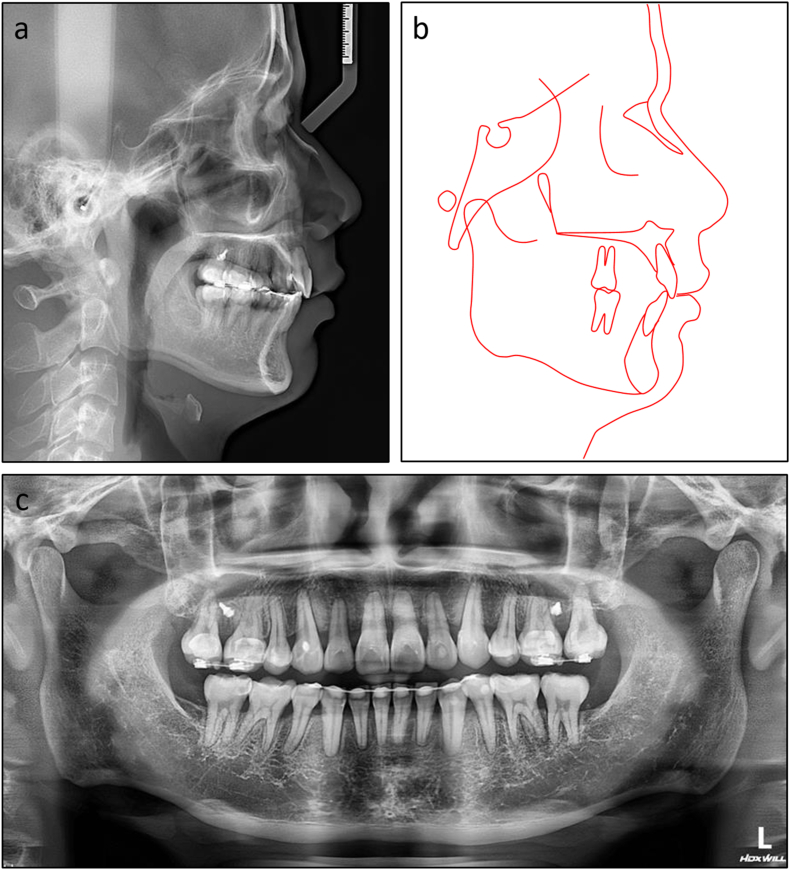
Post-treatment radiographs and cephalometric tracing of Case 2. (a) Cephalometric radiograph. (b) Cephalometric tracing. (c) Panoramic radiograph.
